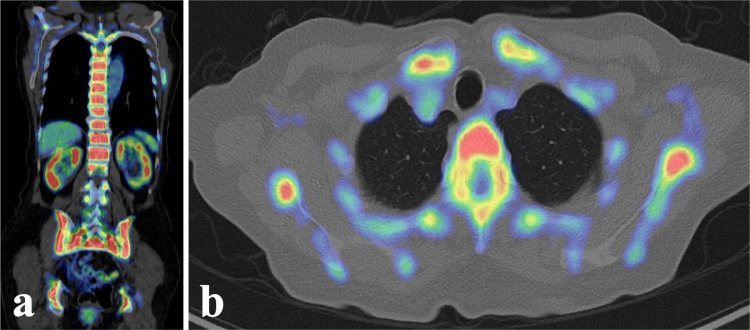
FDG-PET/CT after the fourth SRT (a) Coronal view, (b) Axial view FDG; 2-deoxy-2-(18F) fluoro-D-glucose, PET/CT; positron emission tomography/computed tomography, SRT; stereotactic radiotherapy
